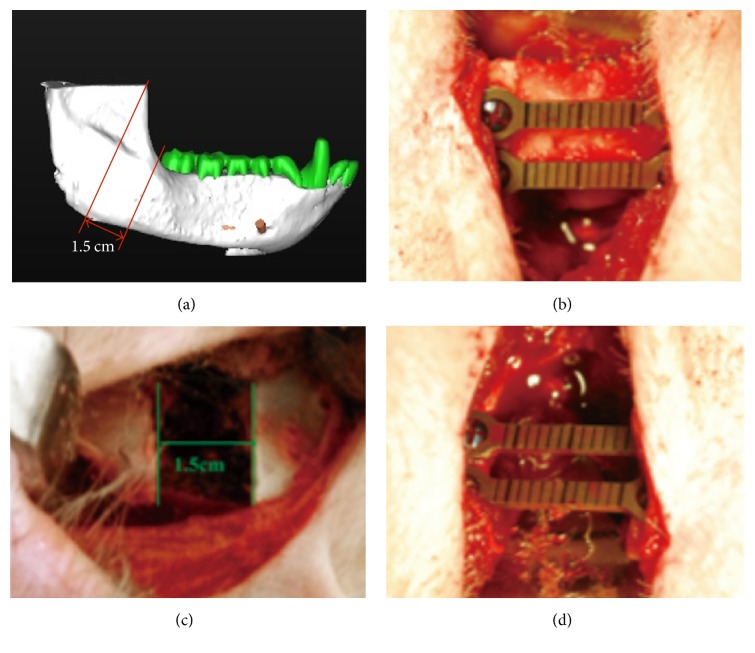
Representative clinical and 3D-rendered imaging volume of mandibular continuity defects. Preoperative CBCT images (a) were used to aid in planning of the segmental osteotomy, as well as in comparison with postsurgical images. Clinical view, showing the surgically exposed posterior mandible, where the reconstruction plates were provisionally attached to the mandible (b). This step defined the position of the titanium screws and reconstruction plates relative to the planned osteotomy to aid in orienting the segmented ends following resection back in correct position. Resected segmental osteotomy, creating a 1.5 cm continuity defect (c). Repositioned mandibular segments fixated with titanium reconstruction plates (d).
